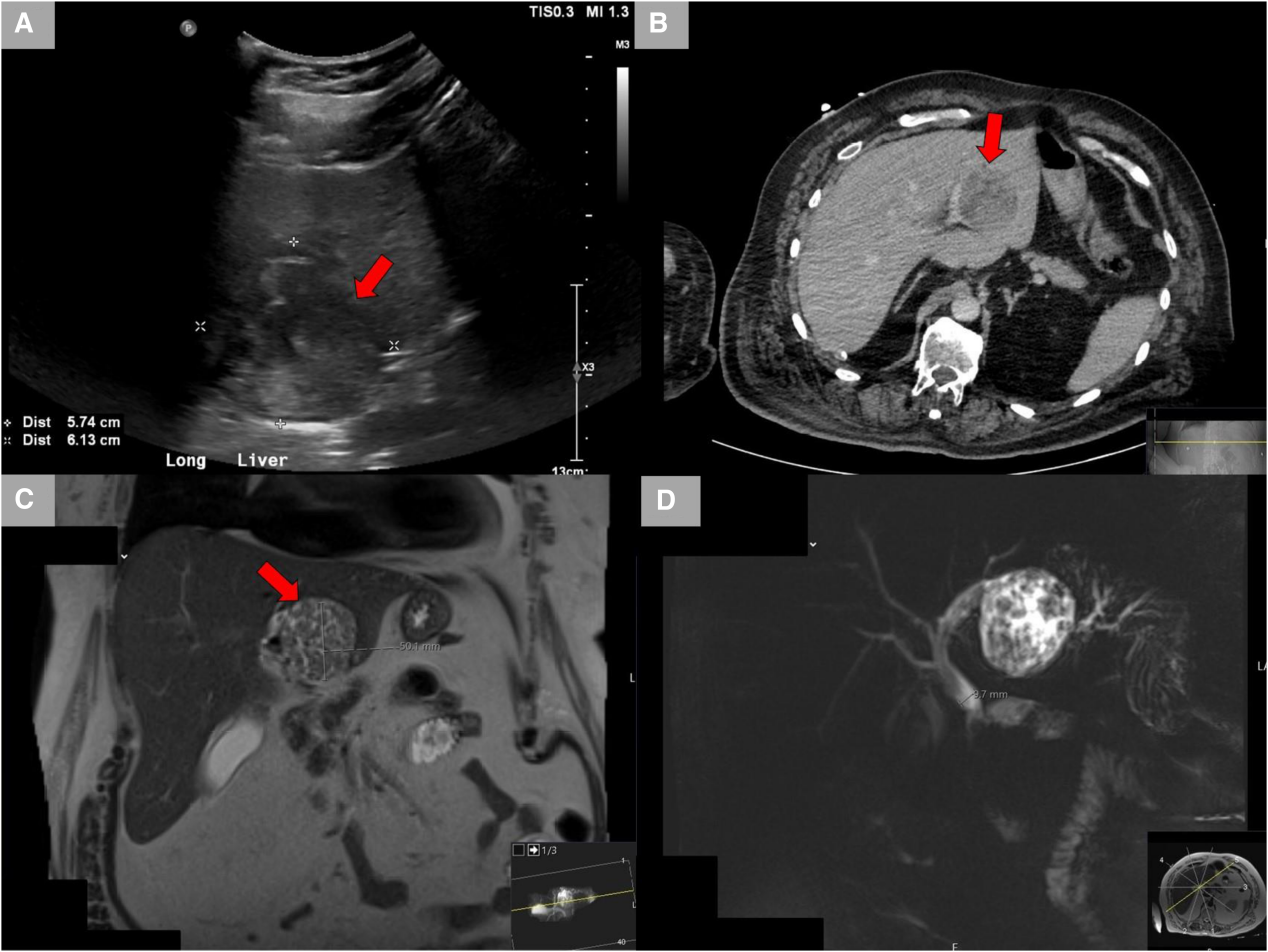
Radiological studies. (A) Ultrasound showing the left hepatic lobe heterogeneous area. (B) CT scan with contrast showing a lesion in the left lobe of the liver demonstrates areas of internal enhancement and a mildly distended gall bladder. (C) MRCP showing a lesion in the left hepatic lobe measuring up to 5 cm. (D) MRCP showing mild gall bladder wall edema without additional signs of cholecystitis and mild CBD dilatation of 9 mm without evidence of choledocholithiasis. CT, computed tomography; MRCP, magnetic resonance cholangiopancreatography; CBD, common bile duct.
